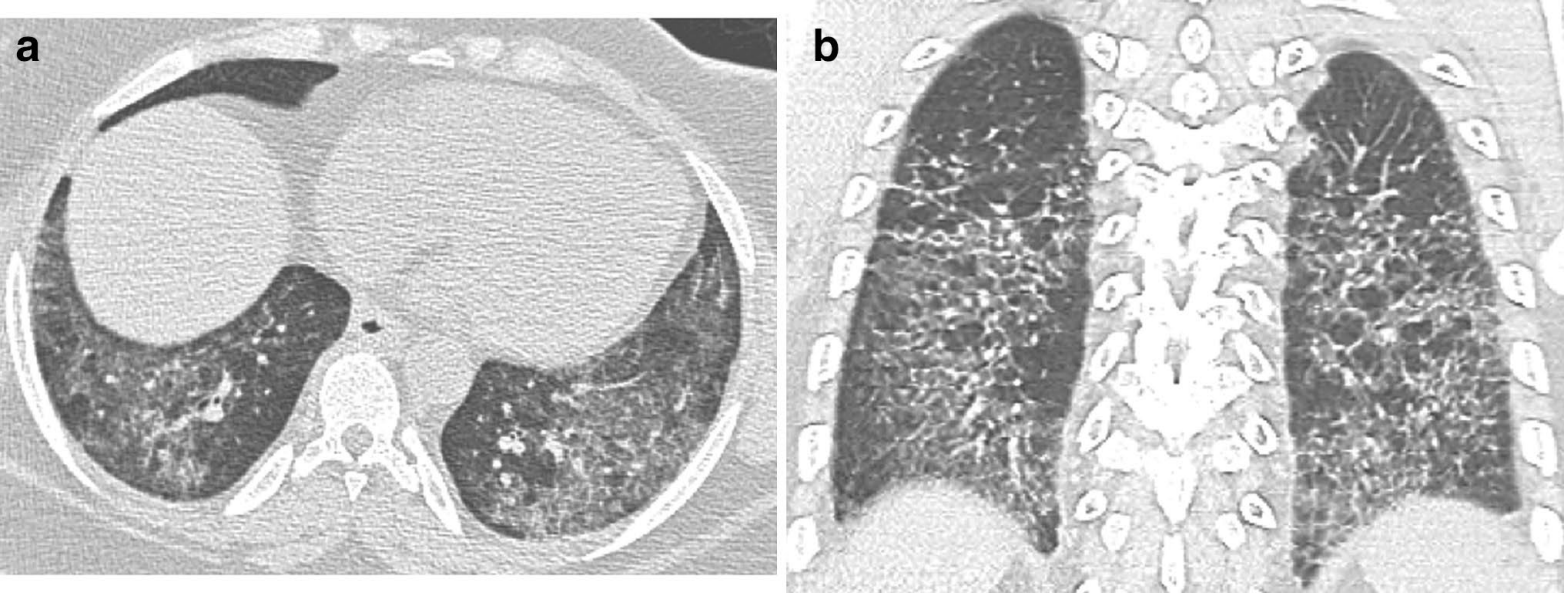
Axial (a) and coronal (b) HRCT images show basilar predominant ground-glass opacity, mild reticulation, mild traction bronchiectasis, and areas of relative subpleural sparing in the lower lobes. These findings are highly suggestive of non-specific interstitial pneumonia (NSIP)
